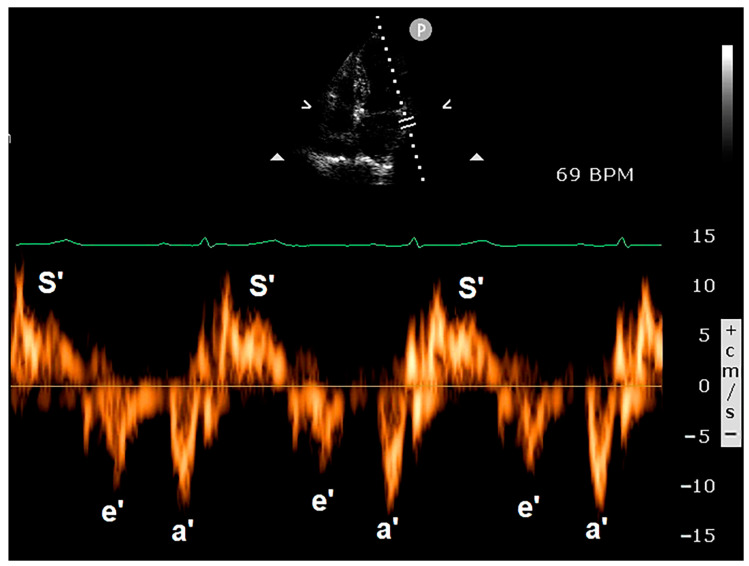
Spectral PW-TDI was obtained by placing the sample volume on the basal lateral wall of the left ventricle. The systolic wave (S’ velocity) represents myocardial contraction, while the two negative waves represent early diastolic myocardial relaxation (e’ velocity) and active atrial contraction in late diastole (a’), respectively. PW, pulsed wave; TDI, tissue Doppler imaging.
